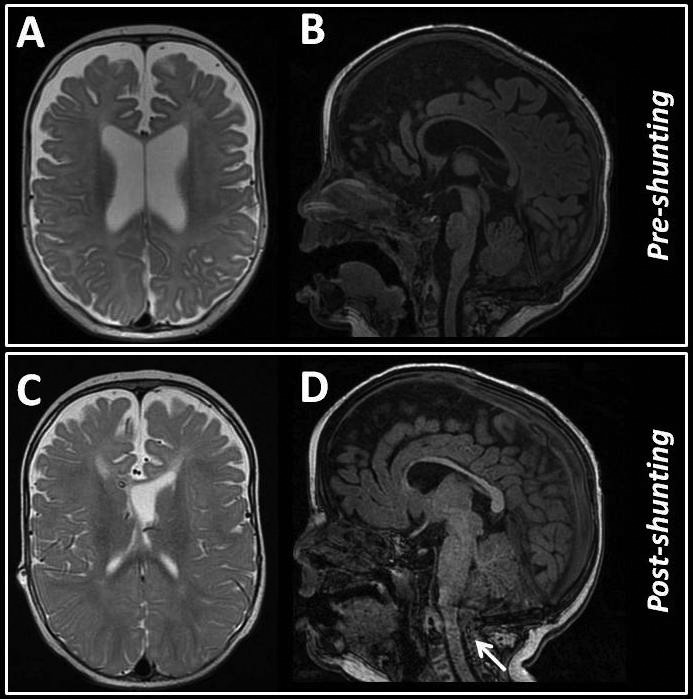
Brain magnetic resonance imaging (MRI) at presentation showed ventricular dilation without apparent obstruction of the cerebrospinal fluid circulation (B),(C). MRI performed two months later ruled out shunt malfunction, but disclosed a crowded posterior cranial fossa with Chiari I malformation (C),(D), arrow.
